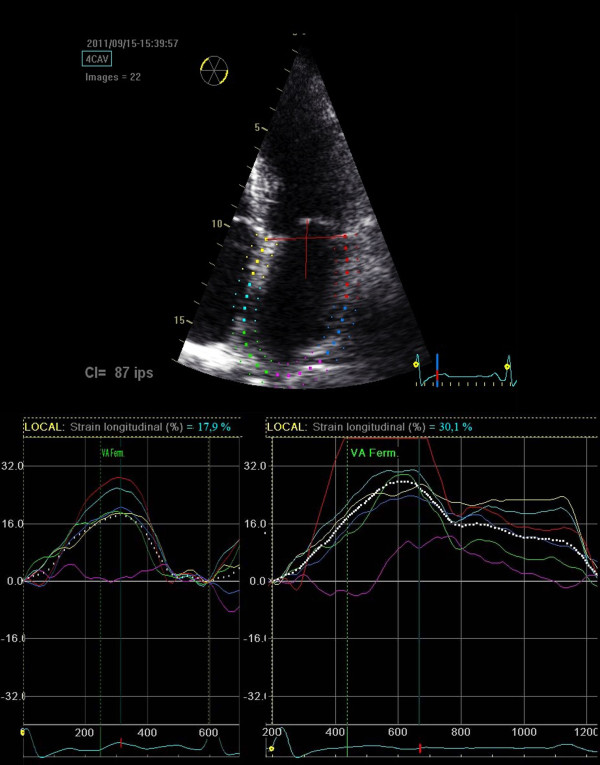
Left atrial speckle-tracking echocardiography. Top. Evaluation of atrial deformation by the speckle-tracking technique. The endocardial contour of the LA is traced in a 4-chamber view. The thickness of the region of interest is then defined. The software automatically tracks atrial wall movements, allowing fine adjustments and verification in real time. Bottom. Strain curves for the same patient as in top figure, examined during AF (left) and after 1 month of SR (right). Each curve represents one of the six LA wall segments; the dotted line indicates the mean strain.
